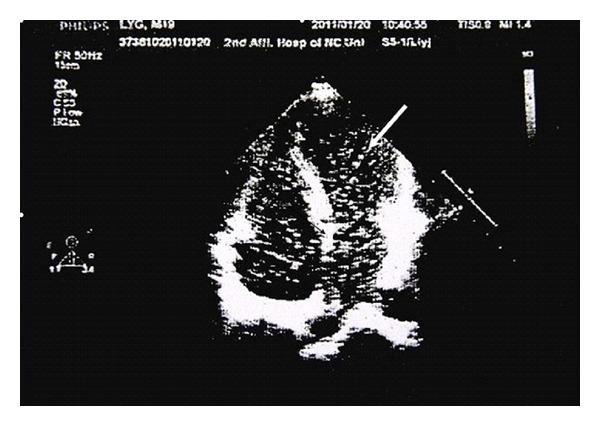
Contrast echocardiography shows that microbubbles (the arrow) produced by contrast agent enter the left ventricle three heart beats after the right.
